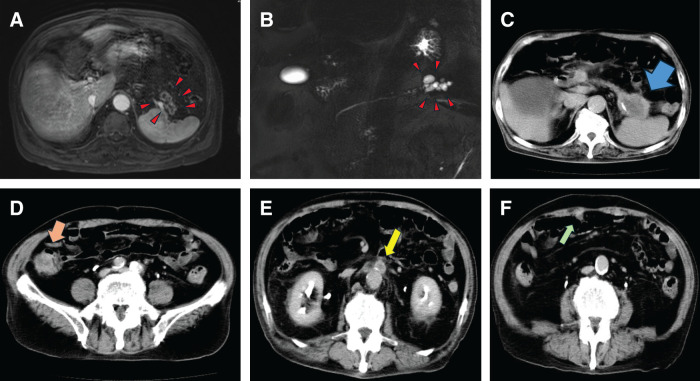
Radiological findings. (A) MRI (e-THRIVE) obtained 4 years before presentation demonstrates a cystic lesion in the pancreatic tail (red arrowheads). (B) MRCP obtained 4 years before presentation shows the cystic lesion in the pancreatic tail (red arrowheads). (C–F) Contrast-enhanced CT at presentation reveals a pancreatic tail tumor (blue arrow), colonic tumors (orange arrow), para-aortic lymph node metastasis (yellow arrow), and peritoneal dissemination (green arrow). e-THRIVE, enhanced T1-weighted high-resolution isotropic volume examination; MRCP, magnetic resonance cholangiopancreatography
